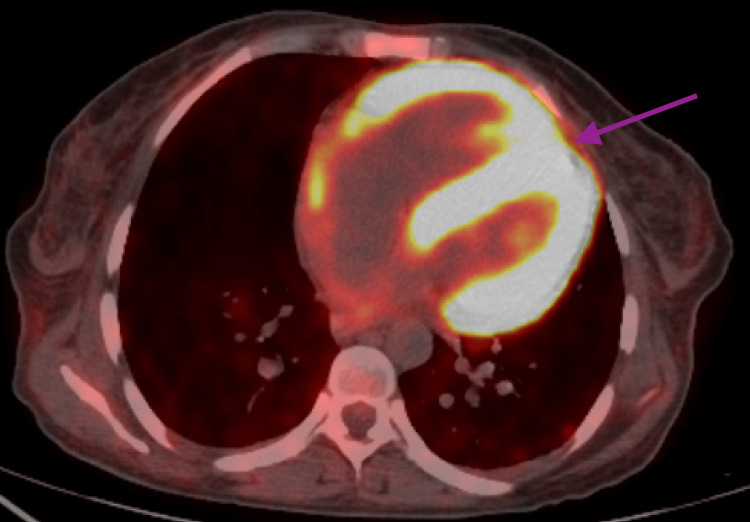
Intense FDG uptake in the cardiac cavities on PET imaging FDG, fluorodeoxyglucose; PET, positron emission tomography
